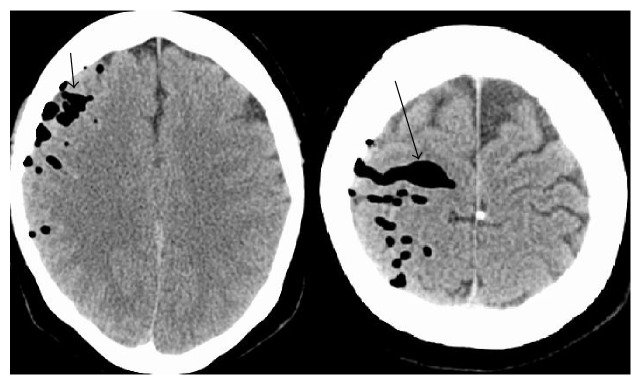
Axial Computed Tomography images of the brain showing air within the right cerebral hemisphere (arrows).
